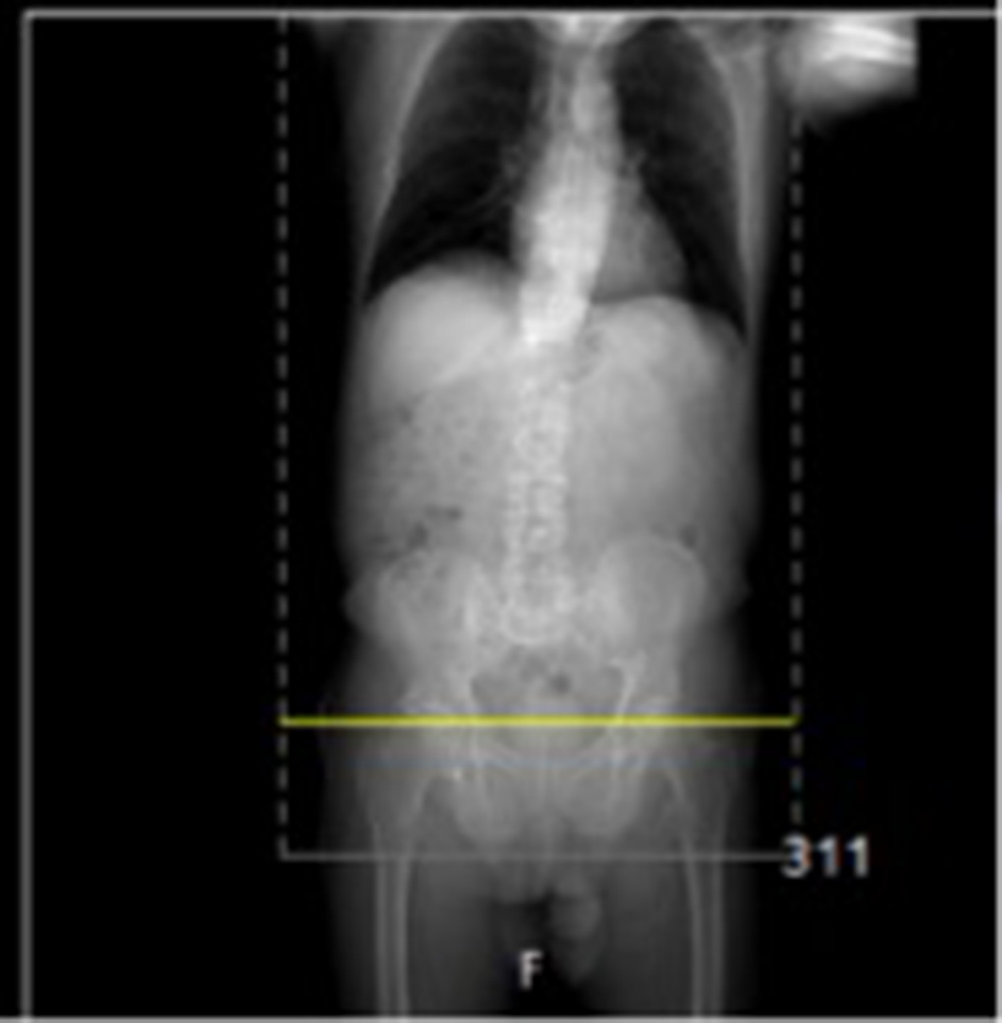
The CT scout view shows no radiopaque foreign body.
